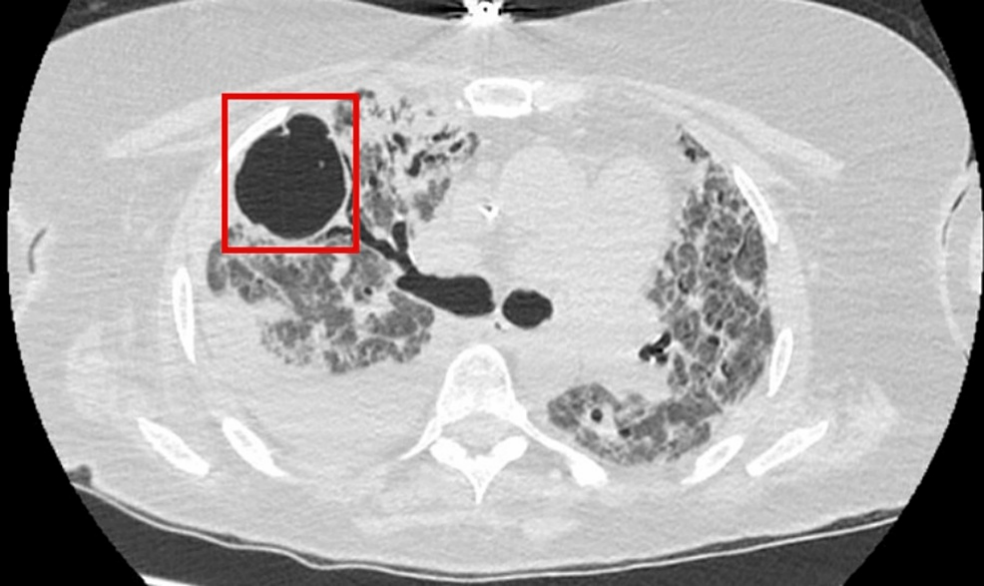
CT thorax showing 5 cm air-filled cyst (red box). Of note, right-sided pleural effusion continues to be present.
